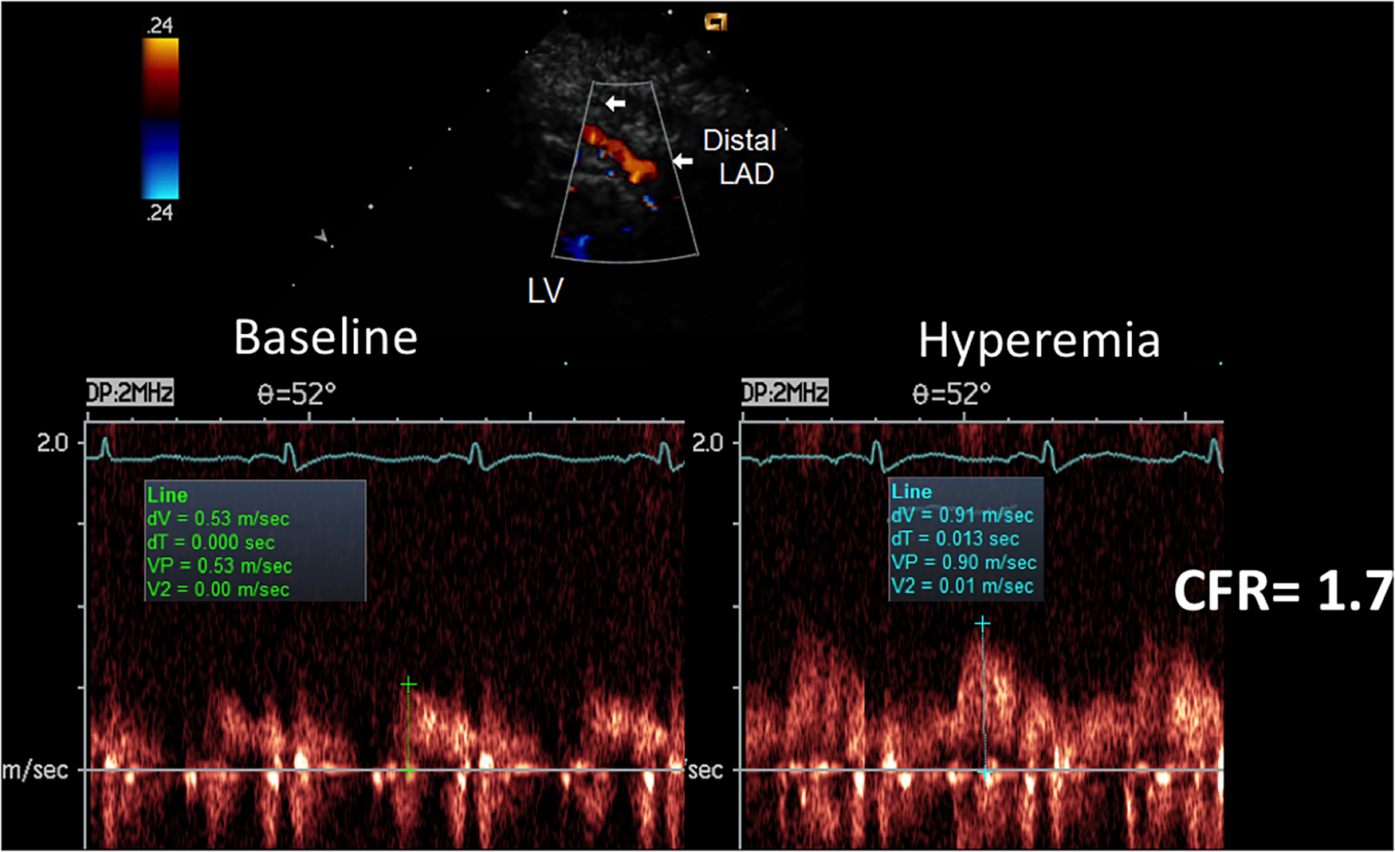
CFR in the distal LAD by E-Doppler TTE in a patient with a subcritical/diffuse LAD athero as assessed by angio. Same layout as the other CFR example. The CFR (peak hyperemic diastolic velocity/peak resting diastolic velocity) is blunted (1.7). The S/D ratio is normal; S/D, ratio of the systolic to diastolic waves of coronary flow velocity.
